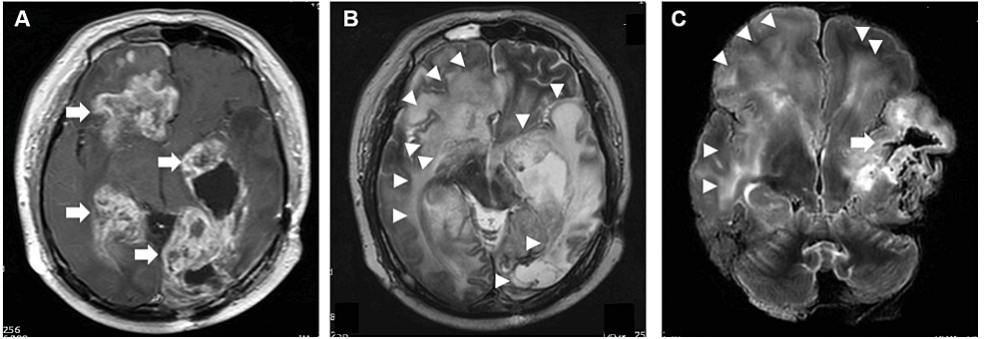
Comparison of the final premortem and postmortem MRI findings in a patient diagnosed with glioblastoma (Case 1) A: T1 contrast image of the final premortem MRI scan showing multiple contrast-enhanced lesions (arrows). B: T2-weighted image of the final premortem MRI showing that T2 high intensity expanded throughout the brain (arrowheads). C: Postmortem T2-weighted images showing T2 high-intensity expansion throughout the brain (arrowheads) and disruptive changes in the brain (arrows).
